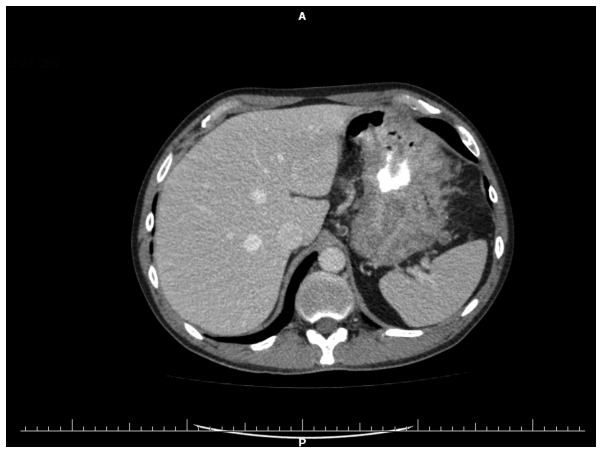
Multi-slice computed tomography examination of the stomach demonstrating the corpus and the prepyloric part with the heterogeneous wall thickened to 34.5 mm.
